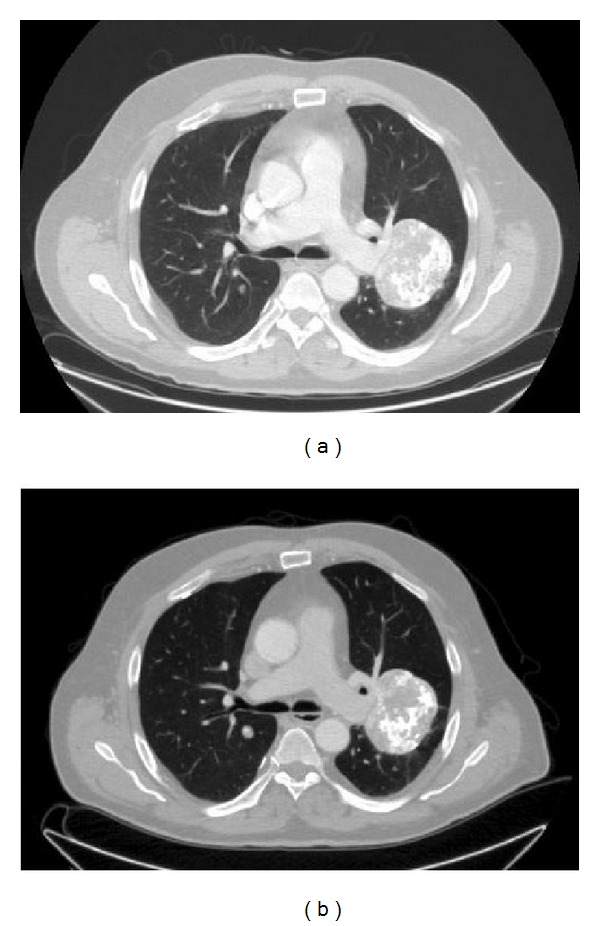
(a) Primary CT from the lung. (b) Control CT from the lung after two and half years of follow-up.
